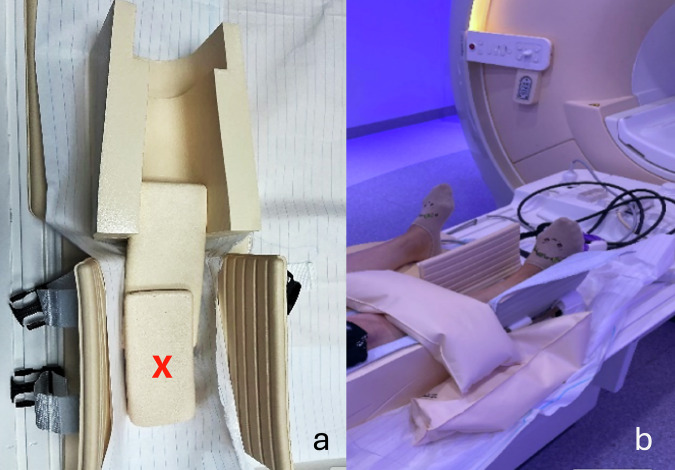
Set up for dynamic MRI of the ankle. a Coil and cushion arrangement; cushion support elevates the lower leg; “X” indicates the position of the ankle joint, placed distal to the center of the coil. b Volunteer in the scanning position; sandbags were placed on and beside the imaged lower leg for stabilization
